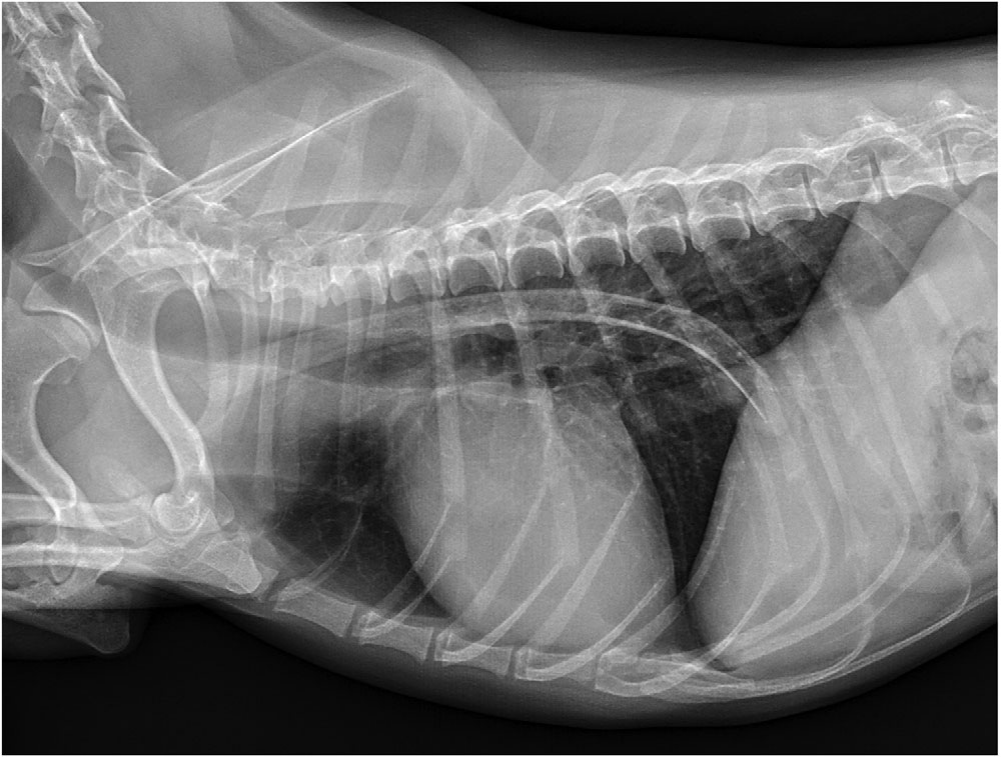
Right lateral thoracic radiography showing a radiodense oesophageal foreign body.
